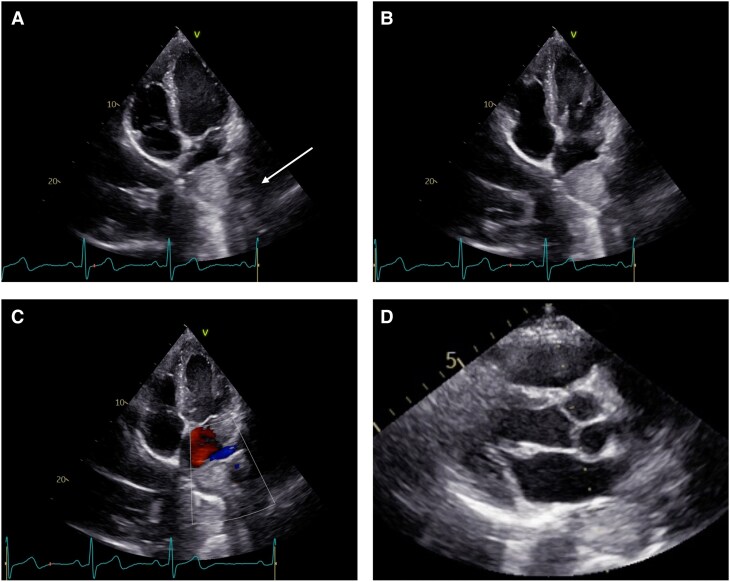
Echocardiographic findings. (*A*) transthoracic apical four-chamber view during tele-diastole showing an echogenic structure within the left atrium (arrow); (*B*) the same view during proto-diastole confirming that the structure compression persists; (*C*) colour Doppler imaging in the same projection showing no flow signal within the structure; (*D*) parasternal long-axis view demonstrating that the structure lies adjacent to, but not within, the left atrial cavity
